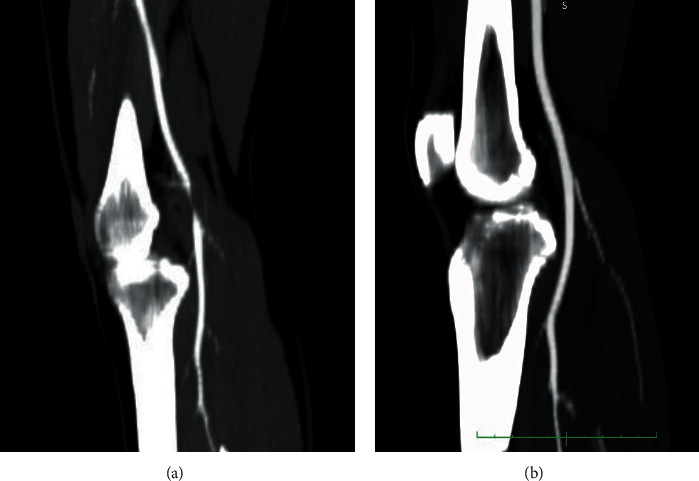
Saggital cut of the CT angiography at the level of the knee: (a) at time of presentation with the arrow showing an obstruction in the right popliteal artery and (b) done at 6 months postpresentation, with no sign of obstruction.
